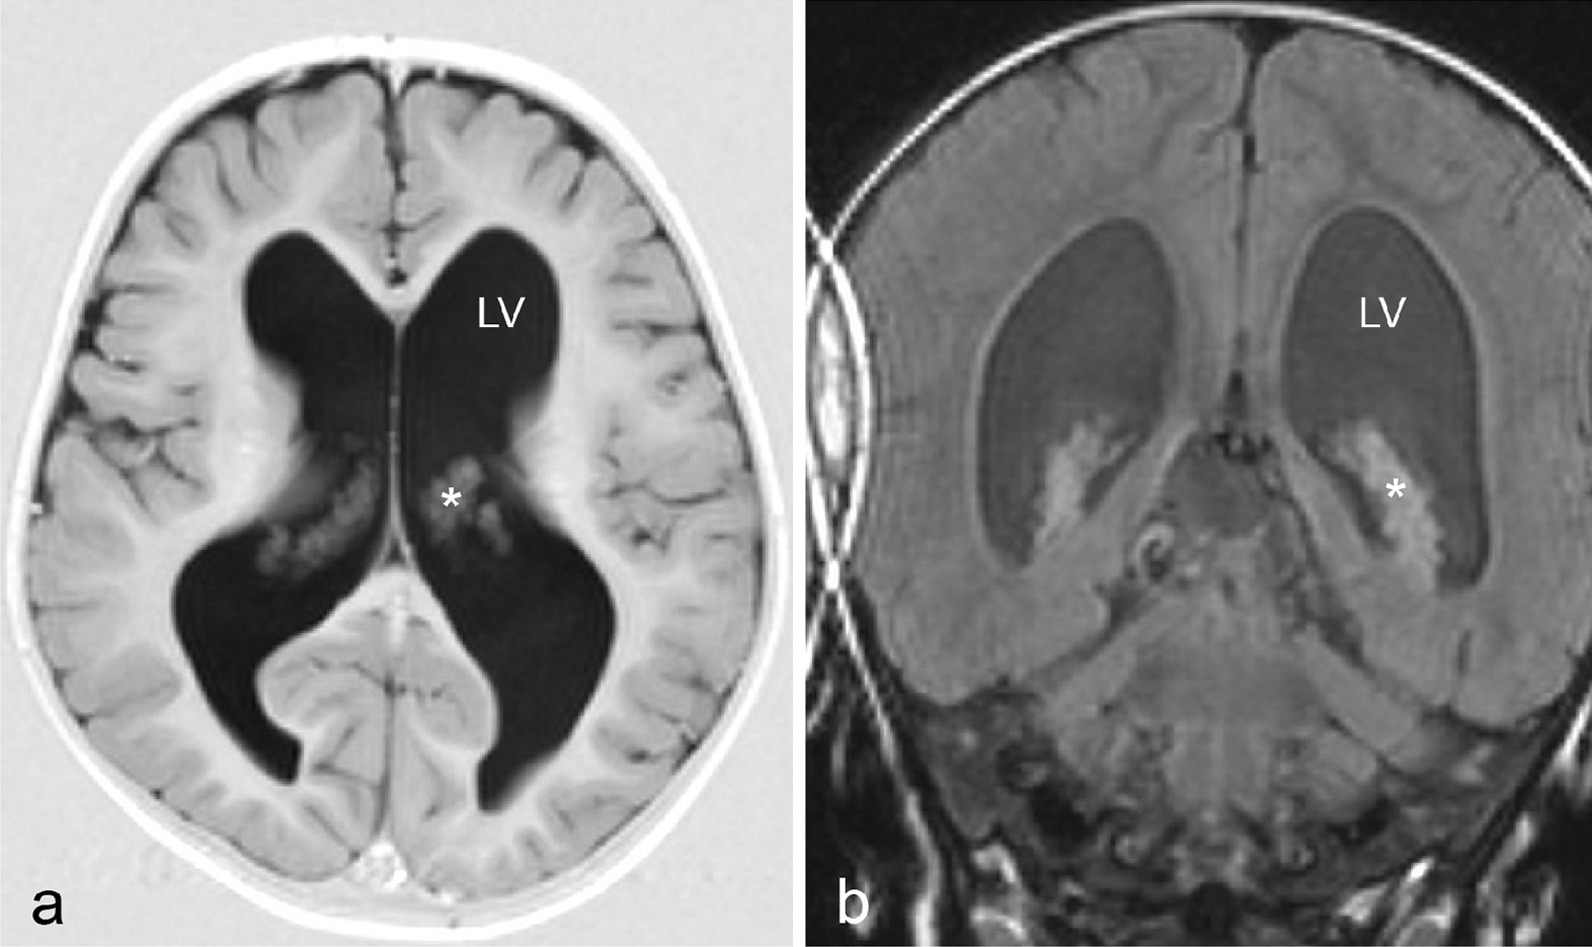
T1-weighted axial (a) and coronal (b) MRI images obtained at patient age 2 years, showing hydrocephalus due to enlarged lateral ventricles (LV) encompassing a prominent choroid plexus (*)
